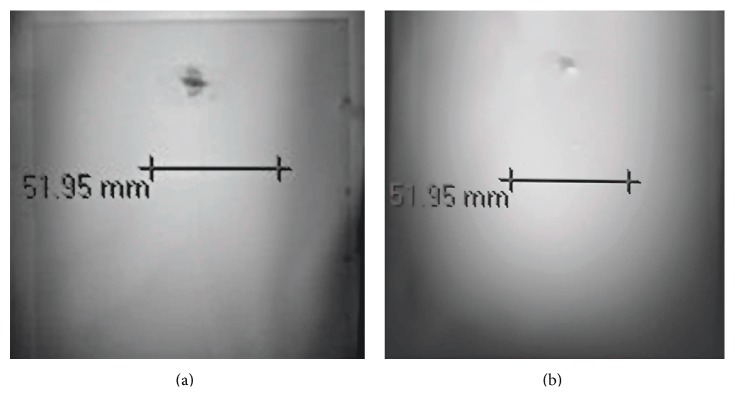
(a) MRI artifact associated with the tissue marker on a gradient echo pulse sequence (TR/TE, 100-msec/15-msec; flip angle, 30 degrees; long axis view; artifact area, 82-mm^2^). The artifact appeared as a low signal intensity signal void that was relatively small in relation to the size and shape of the marker. (b) MRI artifact associated with the tissue marker on a spin echo pulse sequence (TR/TE, 500-msec/20-msec; long axis view; artifact area, 38-mm^2^). The artifact appeared as a low signal intensity signal void that was relatively small in relation to the size and shape of the marker. Note that the artifact size was larger for the gradient echo pulse sequence (a) compared to that observed for the spin echo pulse sequence.
